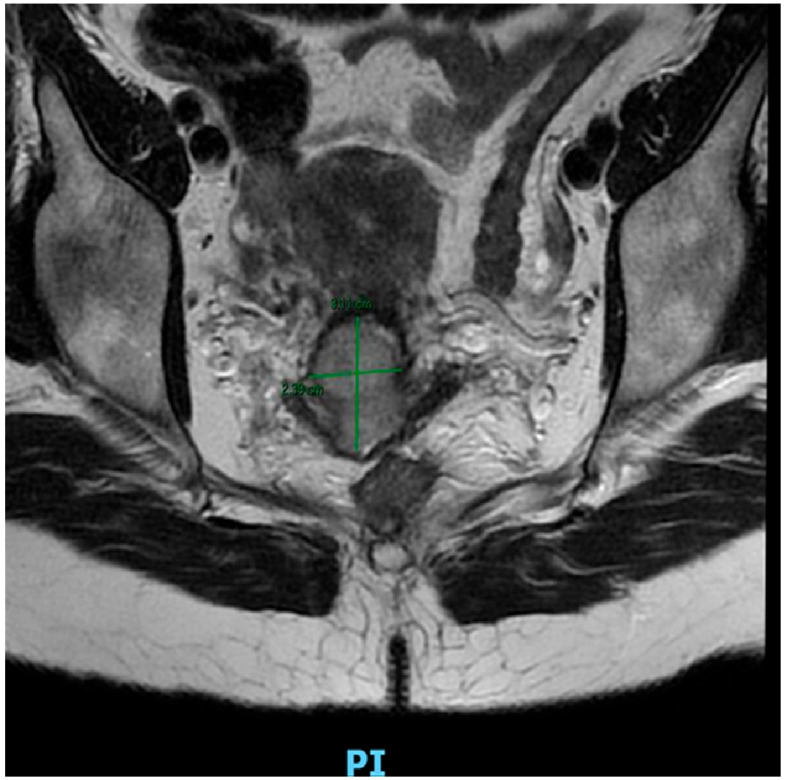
Pelvic MRI (coronal T2-weighted image without contrast) revealing a cervical tumor that measures approximately 3.11 × 2.39 cm, with the lesion appearing hyperintense compared to surrounding cervical stroma, as demonstrated on the images. The lesion is contained to the cervix, which correlates with FIGO stage IB2 cervical carcinoma.
